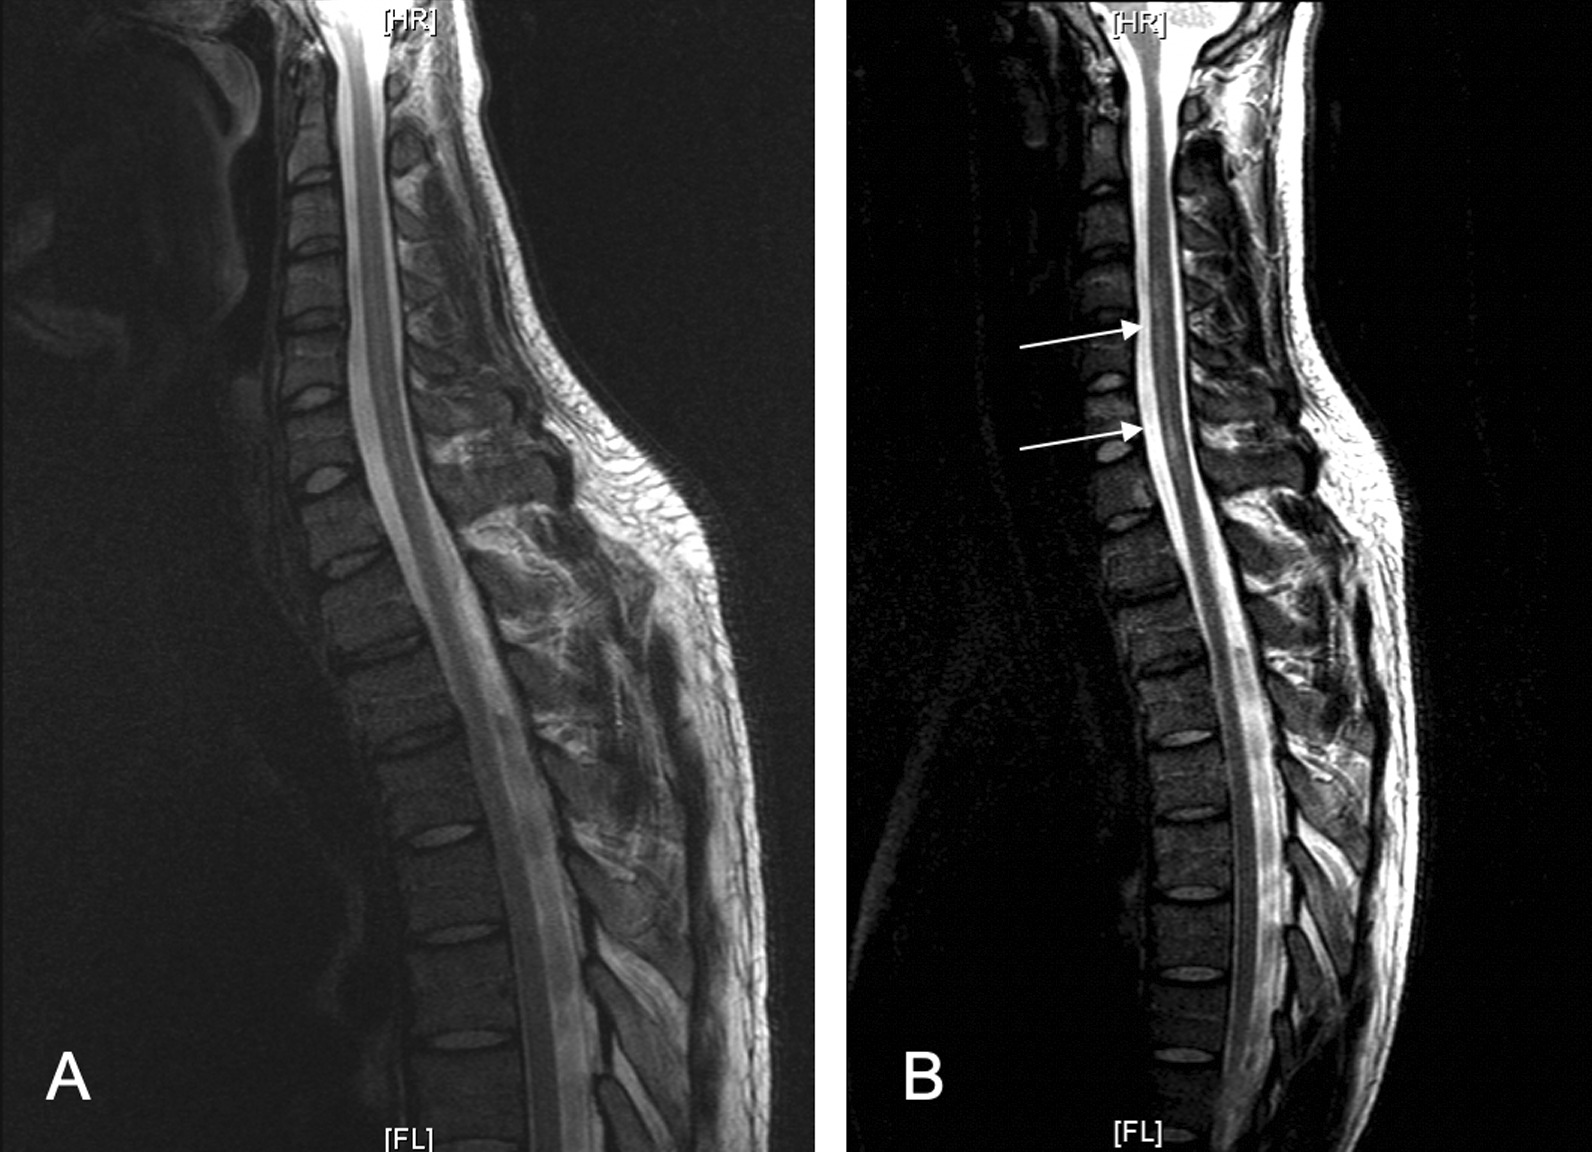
A Initial MRI of the spinal cord, which does not show any lesion. B MRI of the spinal cord 2 days after the onset of symptoms. The sagittal image shows a “pencil-like” hyperintensity extending from C4 to T1 metamer (white arrows)
